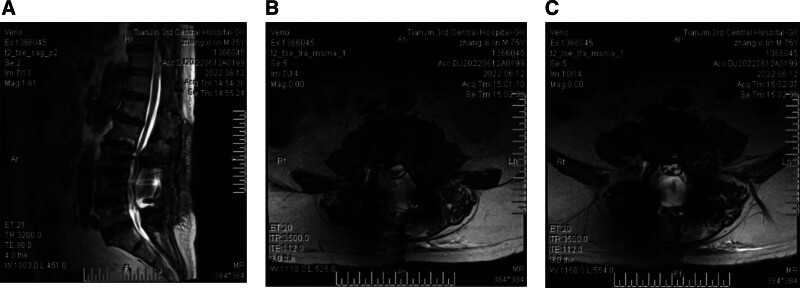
Postoperative MRI showing extradural pseudocyst formation and signs of cauda equina traction. (A) Sagittal T2-weighted image demonstrates a large, well-defined hyperintense lesion posterior to the thecal sac spanning L3 to L5 levels, consistent with an extradural pseudocyst. (B, C) Axial T2-weighted images at the L3 and L5 levels show the pseudocyst occupying the dorsal spinal canal, compressing the thecal sac anteriorly. The cauda equina nerve roots appear clustered, suspended, and disorganized, indicating traction-related changes. MRI = magnetic resonance imaging.
